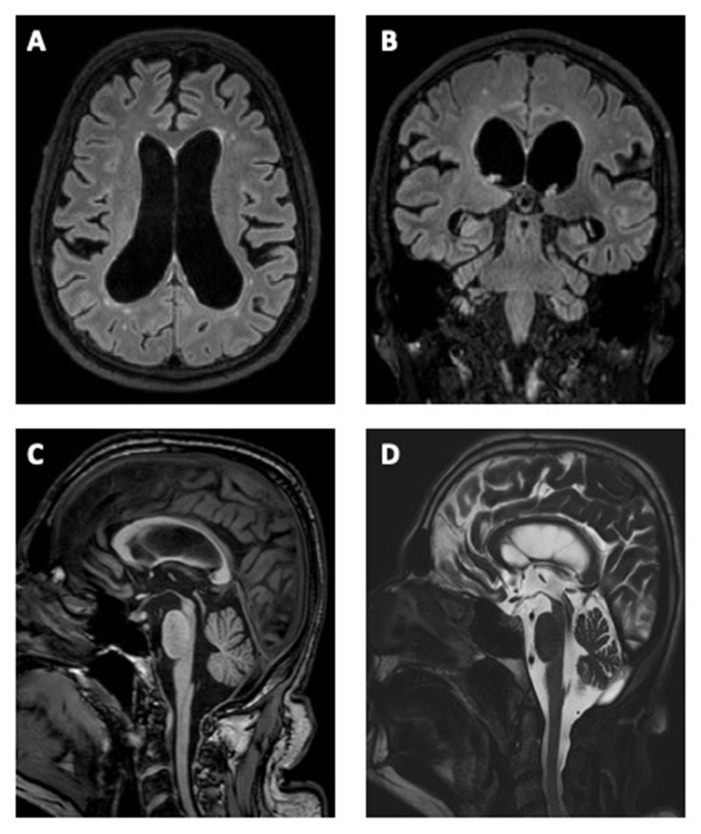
Brain MRI of the typical morphological changes associated with idiopathic normal pressure hydrocephalus and progressive supranuclear palsy. (A) Axial Flair, ventriculomegaly with an Evan’s index of more than 0.3, enlargement of lateral and third ventricles with a widening of the temporal horns, and mild T2 hyperintensities in the periventricular and deep white matter. (B) Coronal Flair, disproportionately enlarged subarachnoid spaces, tight high convexity (narrow sulci and subarachnoid spaces at the vertex and medial/parafalcine region) accompanied by enlargement of the inferior cerebrospinal fluid (CSF) spaces, particularly in the Sylvian fissures. The callosal angle measured at the level of posterior commissure is typically less than 80°. (C) Sagittal T1, midbrain atrophy (penguin or hummingbird sign), thinning and upward bowing of the corpus callosum. (D) Sagittal T2 Drive CSF—midbrain atrophy and strong flow void in the aqueduct of Sylvius.
